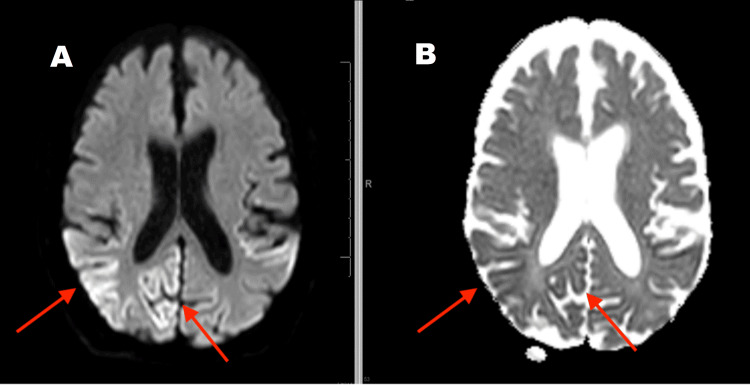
Follow-up MRI Brain after one month shows right occipital region "cortical ribboning" changes (A) Diffusion-weighted imaging (DWI) sequence; (B) Apparent diffusion coefficient (ADC) sequence
